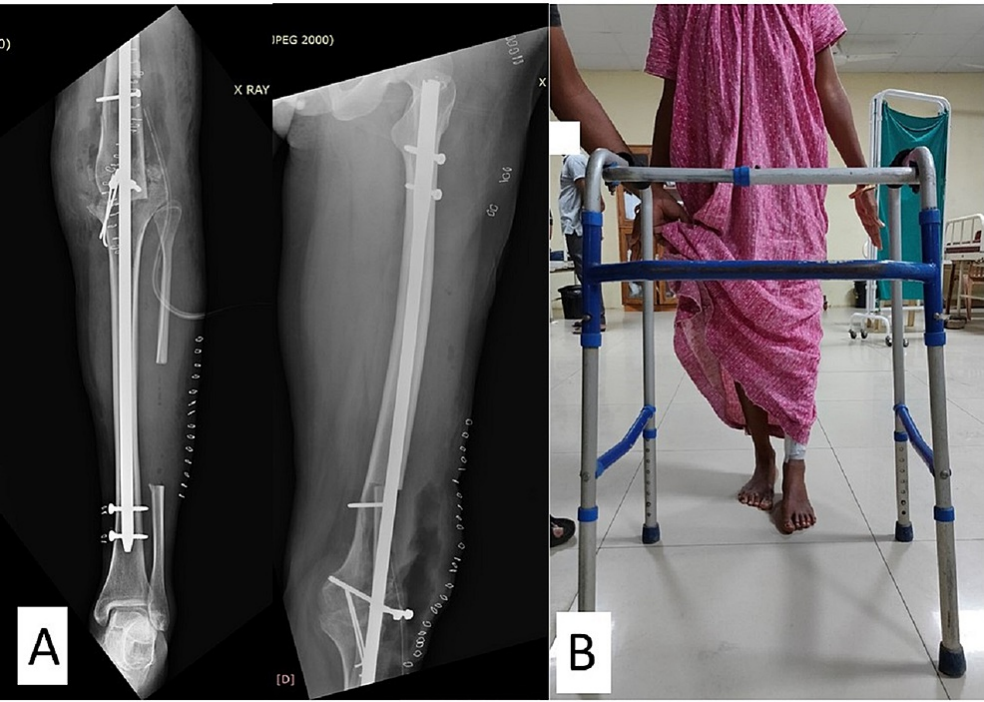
Postoperative radiograph and clinical image (A) Anteroposterior radiograph of left femur and tibia showing nail in situ. (B) Clinical image showing patient using a walker for mobilization.
